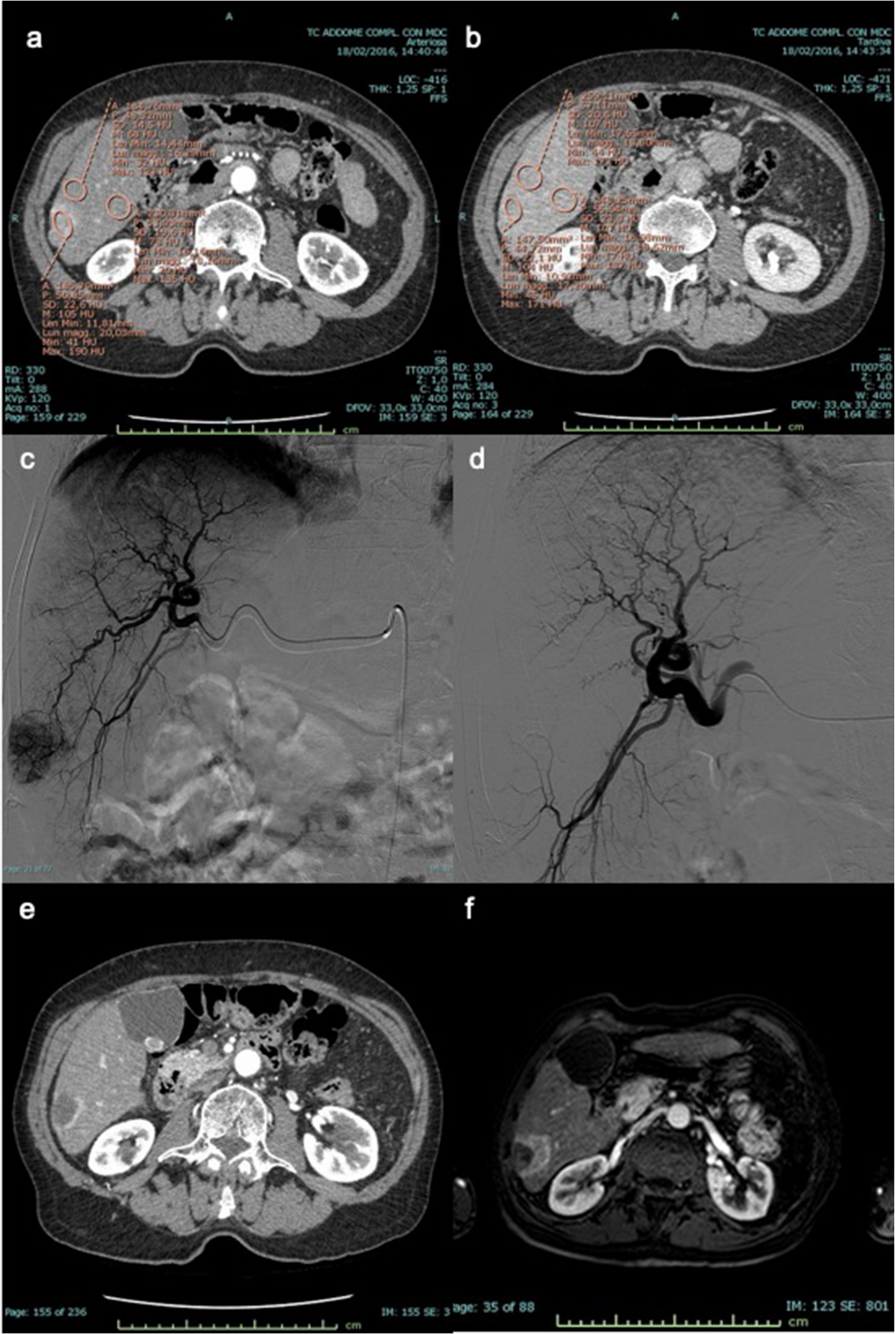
Preprocedural CECT, DSA, and follow-up cross-sectional imaging of an DPAR < 120 and non-smooth margin HCC nodule. For the HCC nodule showed in the example, the following quantitative parameters were calculated (a, b): CER 123.4, LLC 17.4, WO_ab_ 1.7, WO_rel_ 1, and DPAR 102.9. The preliminary DSA evidences a clear hyper-arterialization of the nodule (c), despite relatively low baseline CER and LLC values. While the final angiogram after DEB-TACE shows a complete devascularization of the nodule (d), the 1-month follow-up CECT evidences a residual crescent-shaped area of wash-in on the medial side of the scar (e). The 3-month follow-up MRI scan confirms the presence of obvious residual disease (f)
